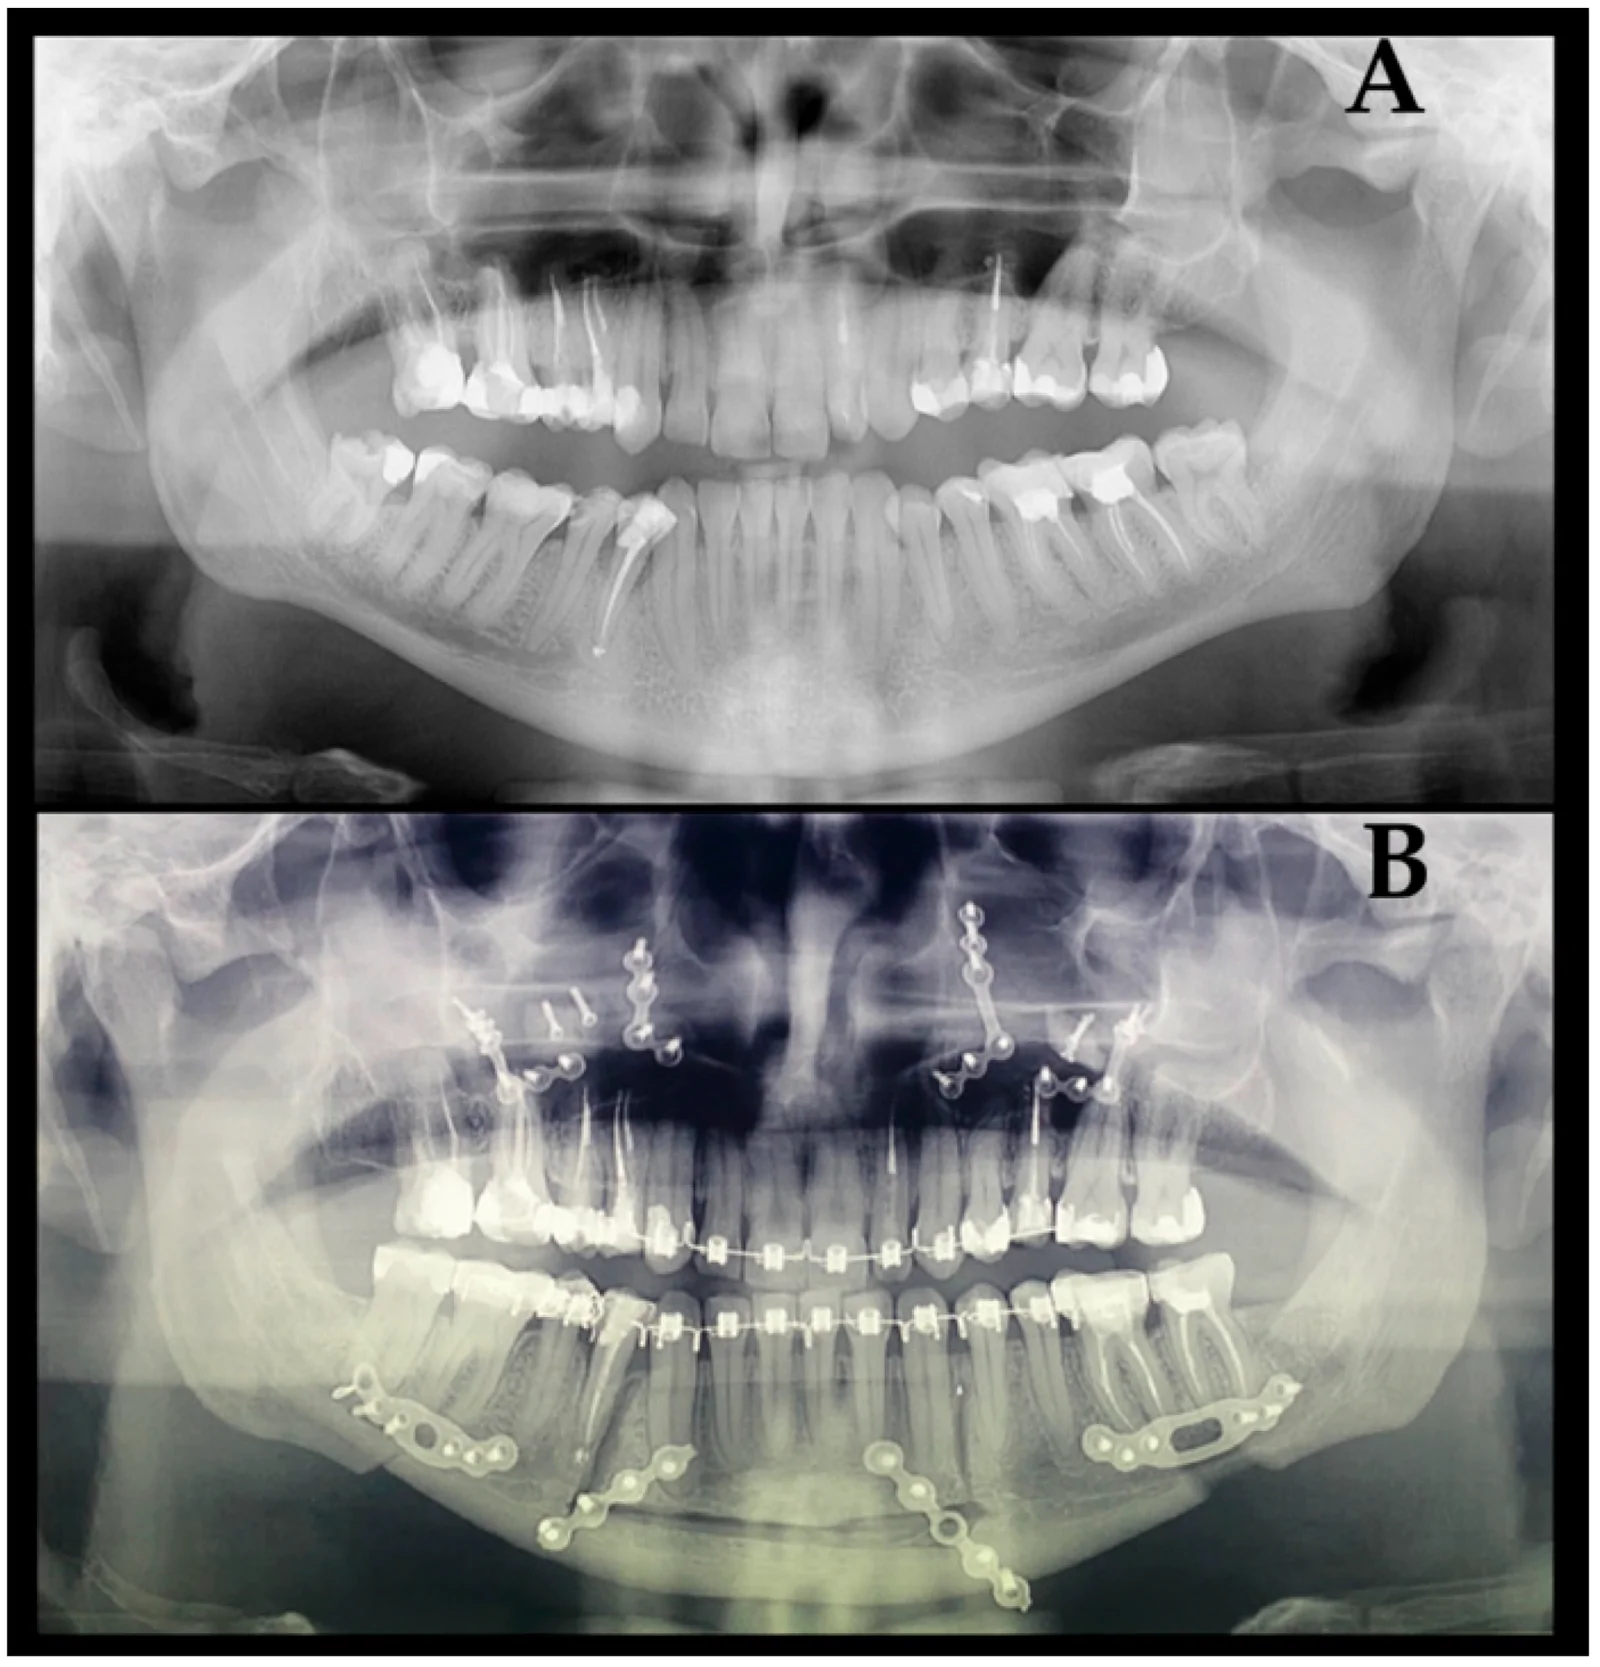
After each surgery, an OPG provides a good, quick, and quite sufficient radiological study to evaluate early outcomes from osteotomies and scope of bone cuts. On the other hand, if any disturbances in bone alignment, condyle head positioning or insufficient radiological appearance of tooth roots and their apices is found, then a CBCT should be done. Initial OPG revealed good radiological outcomes in terms of bone cut placements (A,B), proper bone fragments and osteosynthesis material placement, sufficient central condyle relation in the fossa, and absence of any unwanted bone fractures. Important clinical and radiological considerations for MASSO include changes in tooth angulation and vitality, segment displacement and rotation, bone grafting requirements, and the resulting effects on occlusion, anterior facial height, and the mentolabial region. Additionally, the oval, square, or trigonal-like mandibular and facial appearance can be achieved when the selected mandibular segments are moved, rotated, and positioned in the desired final position. On the other hand, the condition of cortices, status of alveolar bone, presence of good and healthy alveolar bone of at least 1 mm around the dental roots, lack of bone fenestration and bone loss, absence of pre-existing or treatment-related gingival recession (of either orthodontic, periodontal or combined origins), as well as the vitality of the periapical region (dental pulp vitality) and condition of the mental and/or inferior alveolar nerves should be evaluated before surgery. For this reason, CBCT should be considered as a preoperative risk-stratification tool rather than only a diagnostic image, especially when uncommon segmental movements are planned in the anterior mandible. Typical loss of sensation can have various stages, including paresthesia, hyper-/hypo-esthesia, and rarely other neuralgic pain episodes [8,9,10,11]. Surgical perspective should include the type of used burrs and saws to reduce bone overheating and support adequate water-cooling and appropriate plate positions with screws for granting adequate stability, minimizing tooth mobility, and promoting good bone healing [10,11,12,13,14,15,16]. Lastly, the bone segments should also require some steel ligature wiring with the rest of the dental braces/appliances to secure them additionally in the desired position, dental occlusion, and relationship to adjacent teeth, upper incisors, and scope of vertical and horizontal incisal overlapping. Thus, the final treatment plan should combine clinical occlusal evaluation, periodontal assessment, CBCT-based anatomical risk mapping, and intraoperative stabilization principles. Comparison of the preoperative and available early postoperative radiographs demonstrates maintained segment alignment and fixation on routine OPG imaging (A,B). However, while OPG is useful for global postoperative assessment, it cannot replace CBCT for preoperative evaluation of cortical thickness, root proximity, and neurovascular anatomy in complex mandibular subapical osteotomies.
